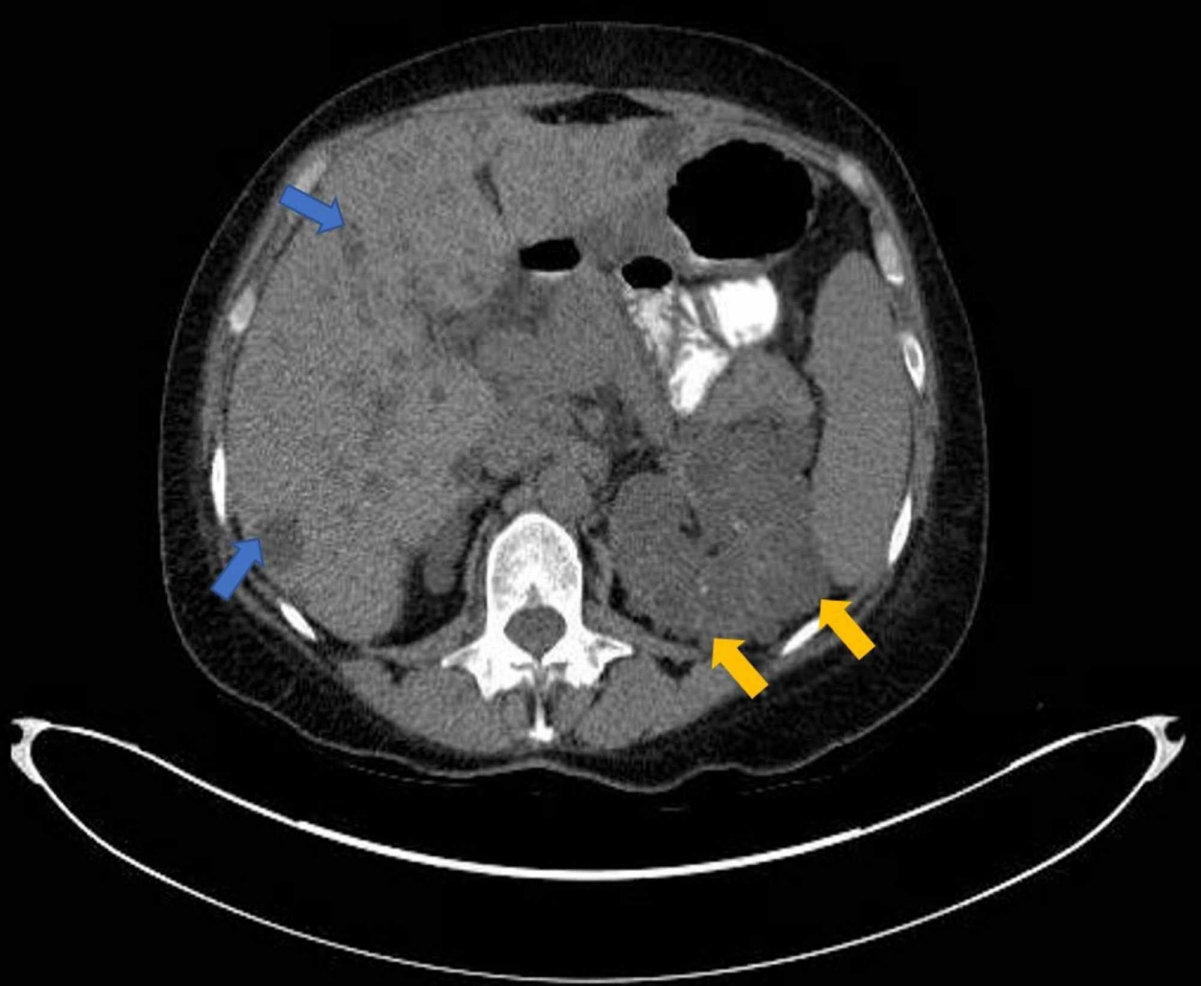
Computed tomography (CT) scan of abdomen and pelvis showing presence of cysts in liver (blue arrows) and cysts in the kidney (yellow arrows)
